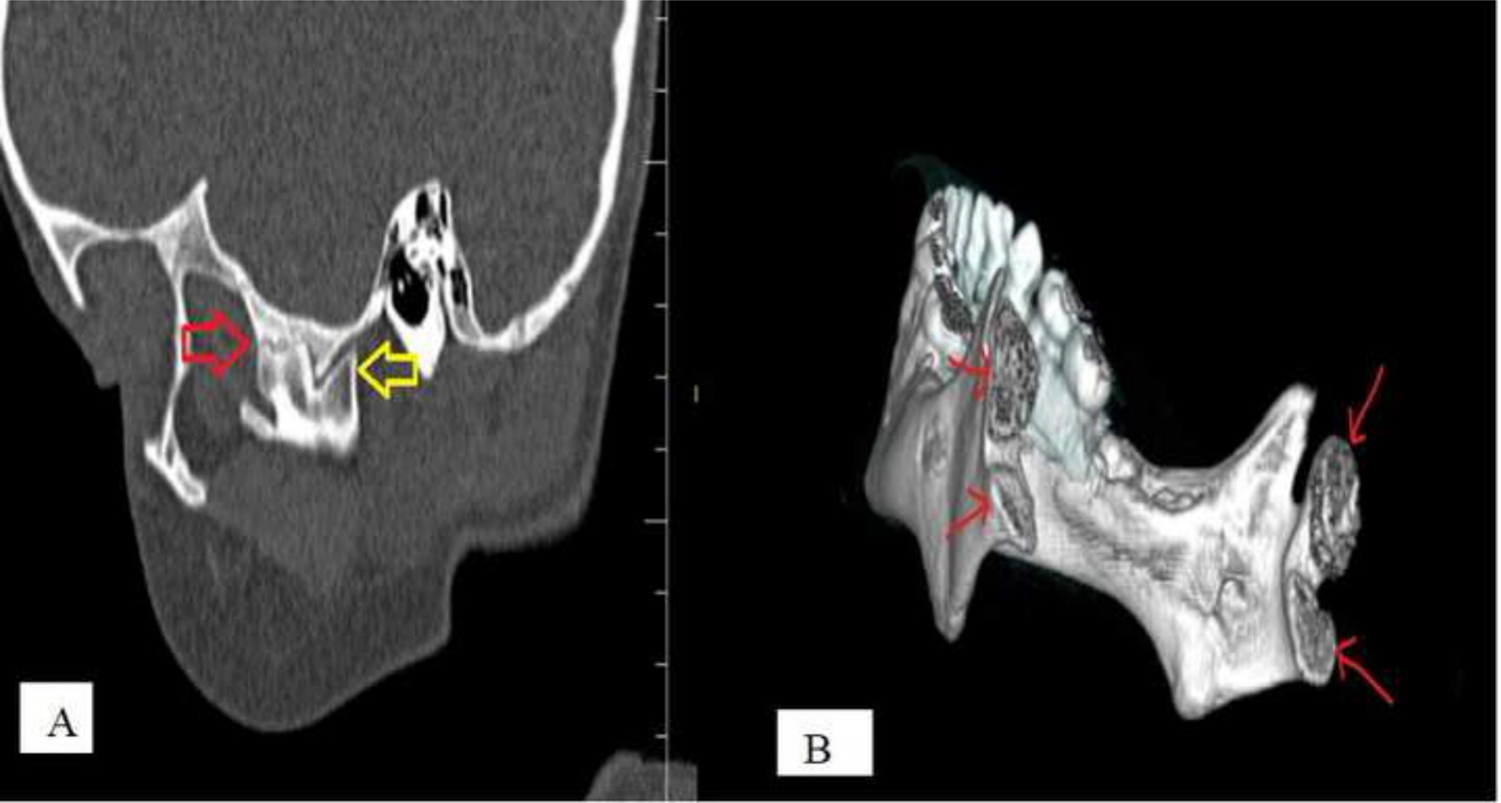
(A) Sagittal reconstructed CT section through the lateral aspect of the left TMJ. The posteriorly placed condylar head is flattened (yellow arrow). The anterior head shows fibrous ankylosis with the temporal bone (red arrow). (B) Three-dimensional reconstructed images show bilateral bifid mandibular condyle (BMC) formation (red arrows).
